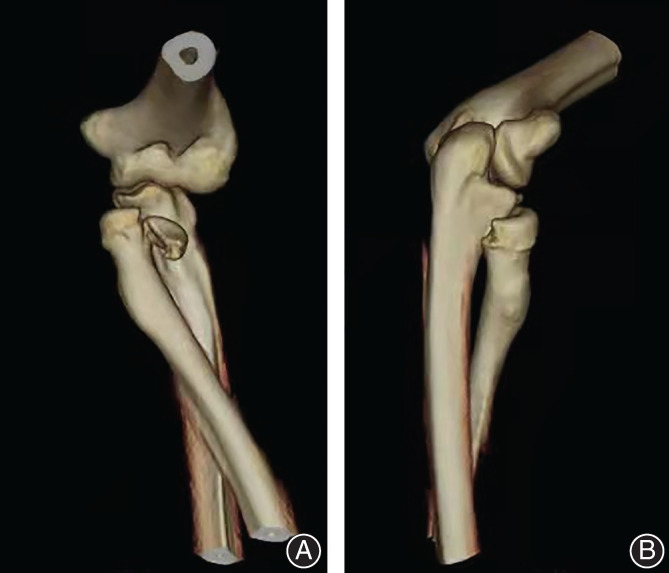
(A) Three‐dimensional CT of the elbow showing dissociation and displacement of the radial head but no dissociation or displacement of the radial neck fracture. (B) Behind the elbow, the radial head rotates the noose to the ulnar tuberosity more clearly
